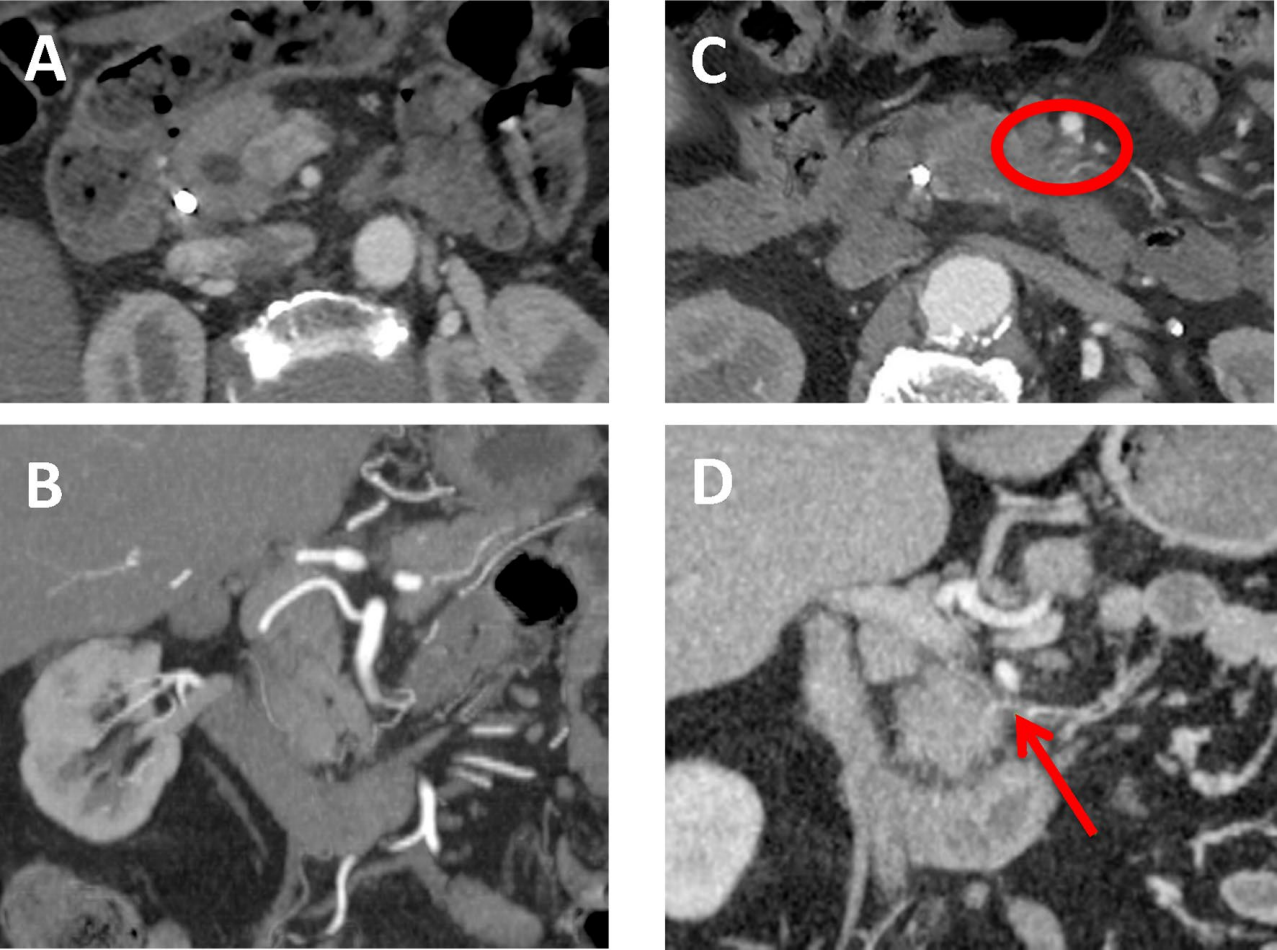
CT-examinations of two different patients without CT-signs of perineural invasion (A,B) and with CT-signs of perineural invasion (C,D) obtained with intravenous contrast material. Axial MIP scans (A) and oblique MIP coronal reformat (B) in arterial phase demonstrate normal fatty tissue surrounding IPDA which suggests the absence of perineural spread. Axial MIP scans (C) and oblique coronal reformat (D) in arterial phase demonstrate the areas with soft tissue attenuation surrounding IPDA (red circle and red arrow), reported as suspicious for perineural invasion.
